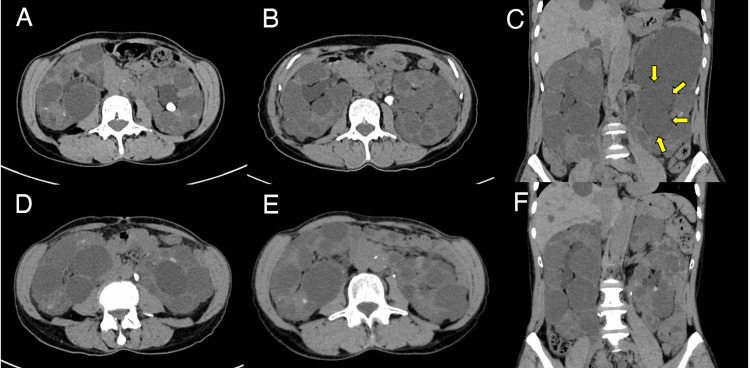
Patient's abdominal CT Abdominal CT revealed (A) a 10.5 mm renal pelvic stone and (B) a 10.3 mm stone in the ureteropelvic junction. CT revealed (C) left hydronephrosis (arrows) and (D) a 9.4 mm ureteric stone at the level of L3/L4. (E and F) A second TUL successfully fragmented and extracted almost all the stone fragments and improved left hydronephrosis. CT: computed tomography, TUL: transurethral lithotripsy
